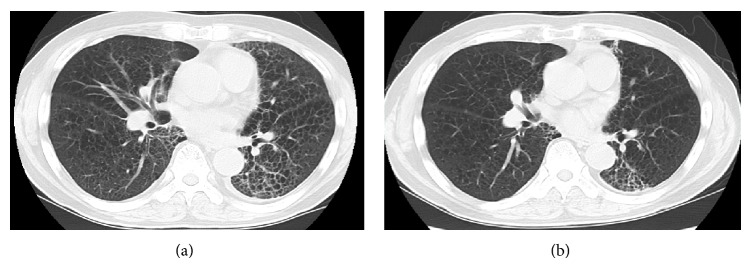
Chest CT scan on admission (a) shows bilateral ground-glass and reticular opacities. Fifteen days after the administration of corticosteroid, bilateral ground-glass opacities reduced (b).
